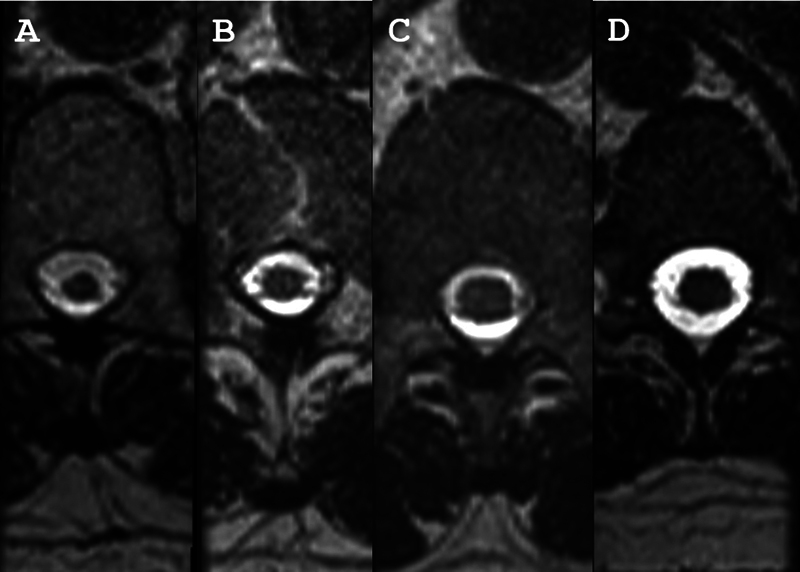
Magnetic resonance imaging scan of the lumbar spine in the axial plane of individuals with definite HAM (
